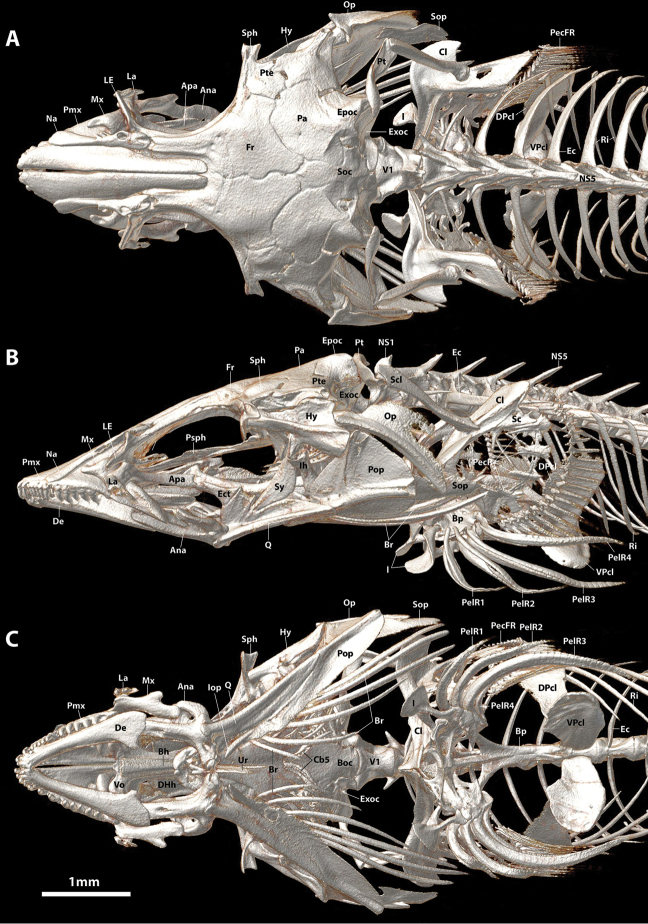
CT scanned anterior skeleton of *Aspasmogastercostata*, TCWC 17166.01, 30.0 mm SL. A Dorsal view B Lateral view (left side) C Ventral view. Abbreviations: ACh, anterior ceratohyal; Ana, anguloarticular; Apa, autopalatine; Boc, basioccipital; Bp, basipterygium; Br, branchiostegal rays; Cb5, ceratobranchial 5; Cl, cleithrum; DHh, dorsal hypohyal; DPcl, dorsal postcleithrum; Ec, epicentral; Ect, ectopterygoid; Epoc, epiotic; Exoc, exoccipital; Fr, frontal; Hy, hyomandibular; I, pelvic-fin spine; Iop, interopercle; La, lachrymal; LE, lateral ethmoid; Na, nasal; NS1, 5, neural spine of vertebral centrum 1, 5; Op, opercle; Pa, parietal; PecR, pectoral radial; PecFR, pectoral-fin ray; PelFR1-4, pelvic-fin ray 1–4; Pop, preopercle; Pro, prootic; Psph, parasphenoid; Pt, posttemporal; Pte, pterotic; Q, quadrate; Ra, retroarticular; Ri, rib; Sc, scapula; Scl, supracleithrum; Soc, supraoccipital; Sop, subopercle; Sph, sphenotic; Ur, urohyal; V1, vertebral centrum 1; Vo, vomer; VPcl, ventral postcleithrum.
